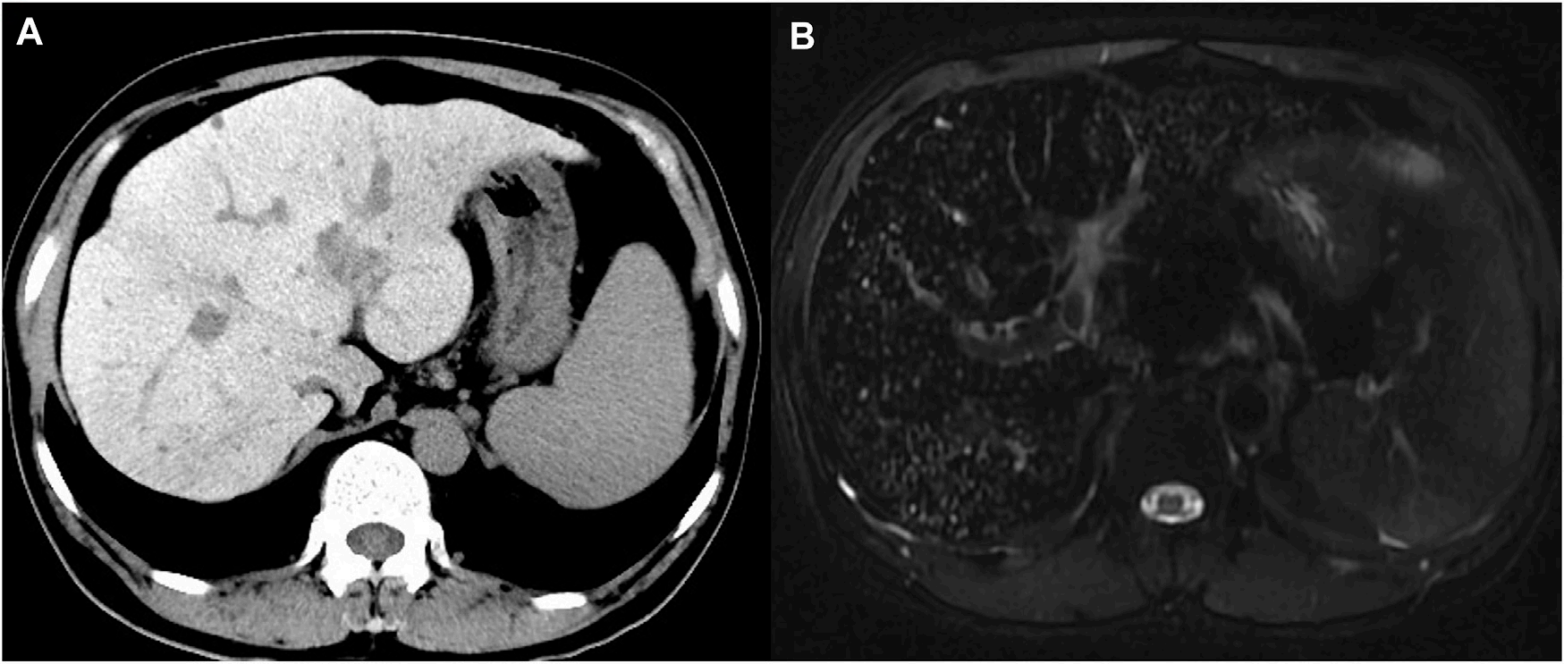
Computed tomography and magnetic resonance imaging of the proband. (A) Precontrast CT showed hyper-attenuation of liver parenchyma; (B) MRI T2-weighted image showed diffuse low signal intensity of liver parenchyma obviously.
